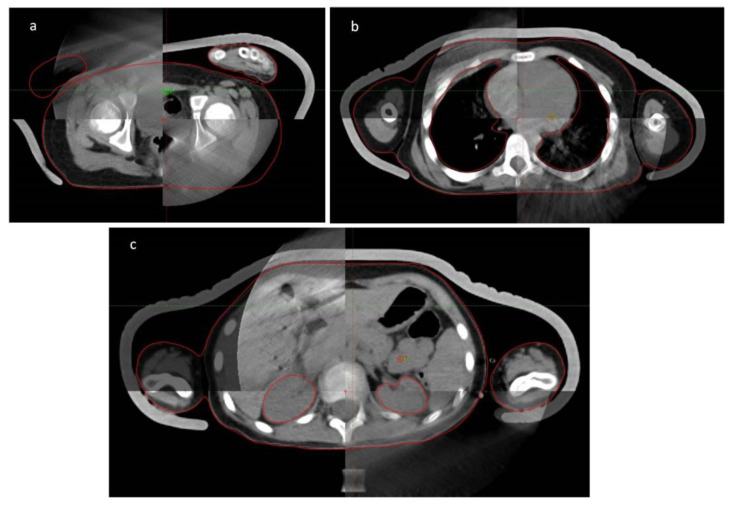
Image guided kilovolt cone-beam CT controls: (a) at the isocenter level (b) at lung area, (c) at kidney area.
